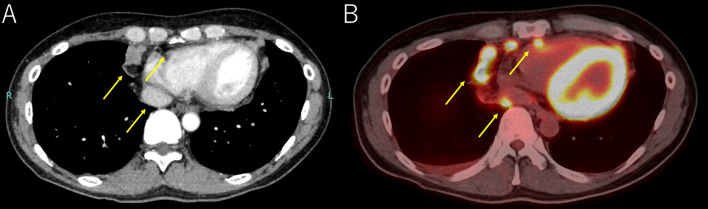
CE-CT (A) and PET–CT (B) images showing apparent bilateral pleural dissemination and para-phrenic lymph node metastasis (arrow)
